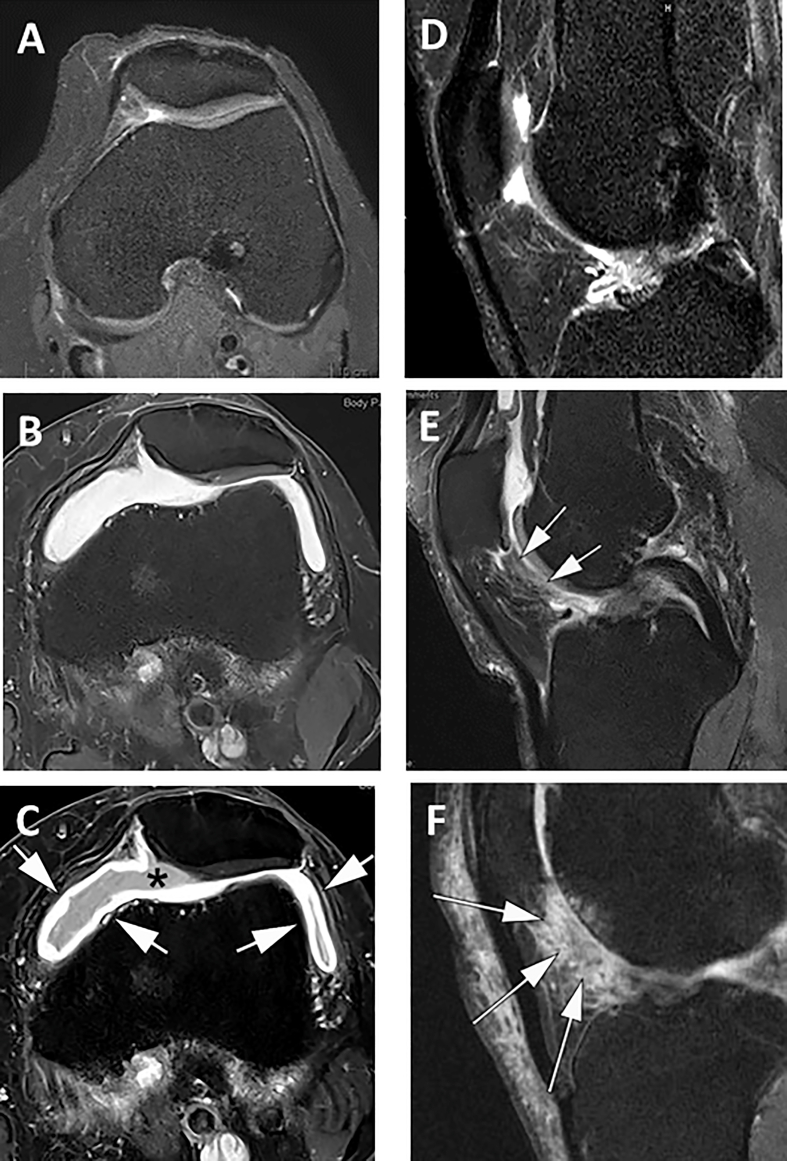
MR imaging of effusion and Hoffa synovitis: A) Axial intermediate-weighted fat suppressed non-enhanced image (3T) not showing any signs of effusion synovitis, B) Axial intermediate-weighted fat suppressed non-enhanced image (3T) depicting severe effusion-synovitis, not differentiating effusion (intra-articular joint fluid) from thickening of the synovial membrane, C) Axial T1-weighted fat suppressed image after i.v. contrast administration (3T) allowing for differentiation of the hypointense joint fluid (asterisk) and the (enhanced) thickened synovial membrane, reflecting synovial hyper-vascularization (arrows), D) Sagittal intermediate-weighted non-enhanced fat-suppressed image (3T) not showing any signs of Hoffa synovitis, E) Sagittal intermediate-weighted fat-suppressed non-enhanced image (3T) showing moderate Hoffa synovitis, most pronounced in the posterior IPFP adjacent to the synovial membrane (arrows), F) Sagittal intermediate-weighted non-enhanced fat-suppressed image (1.5T) displaying severe Hoffa synovitis with marked diffuse signal hyperintensity in the body of the IPFP (arrows). Images kindly provided by Frank Roemer, MD, Universitätsklinikum Erlangen, Germany.
